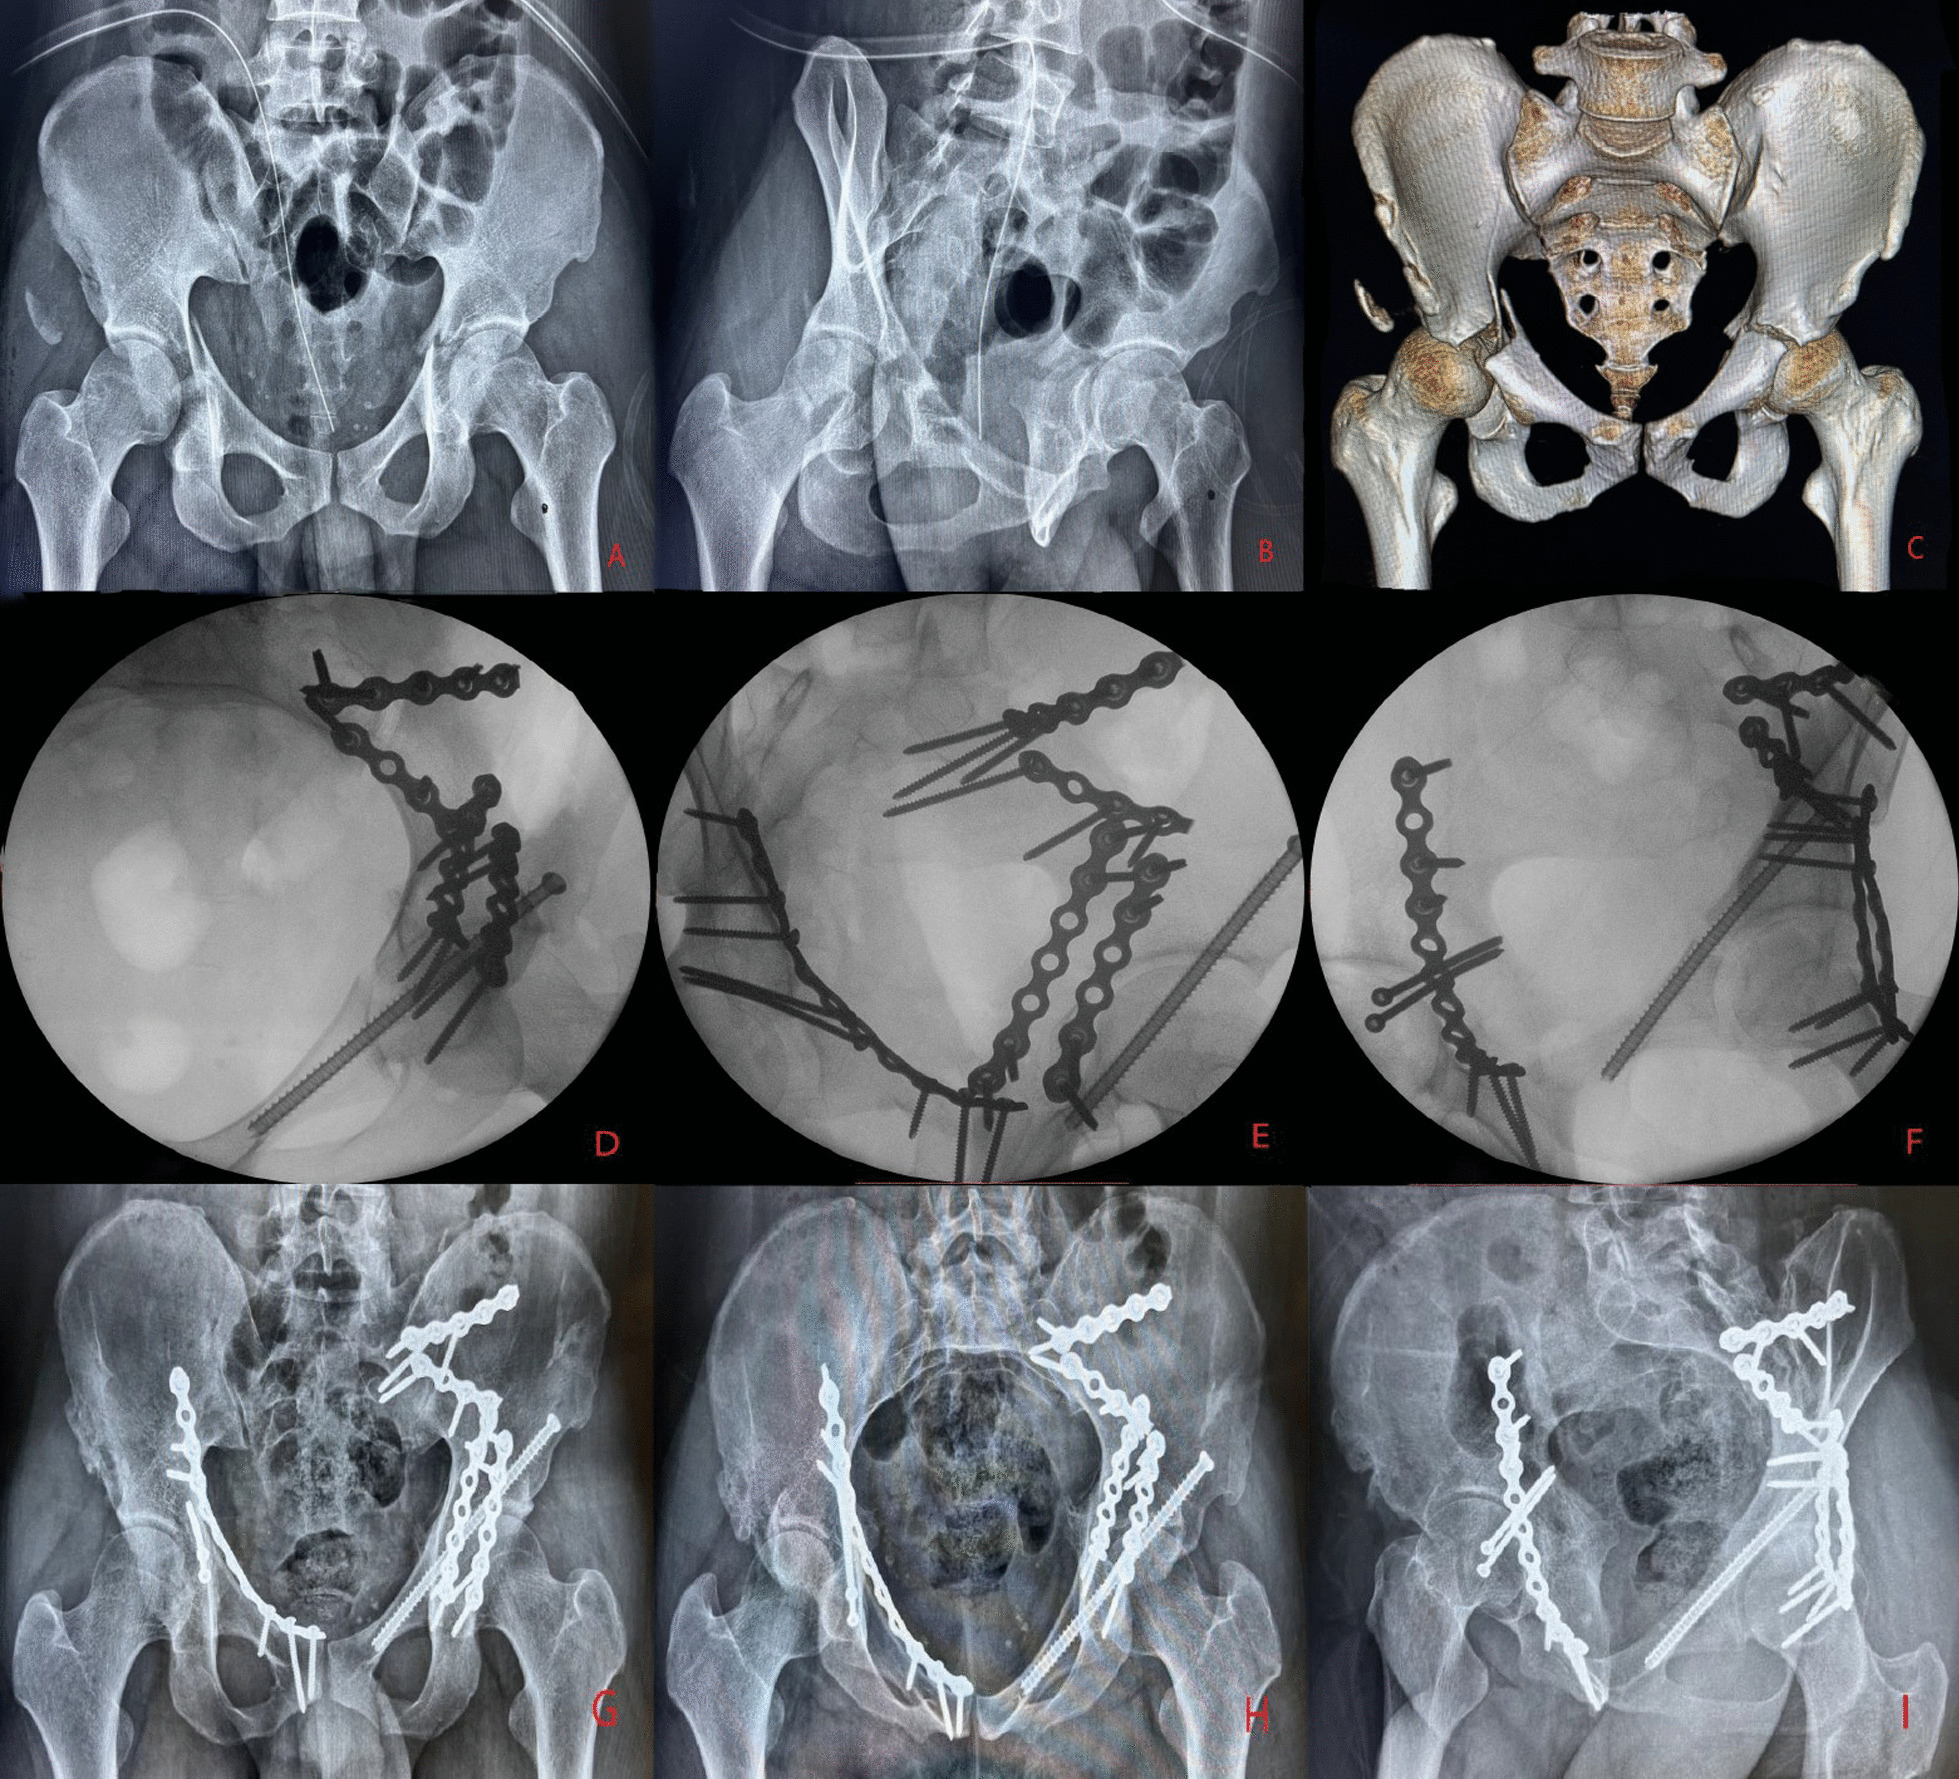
A 25-year-old male patient suffered a Tile C pelvic fracture with bilateral transverse acetabular fractures as a result of a motor vehicle accident and received his first surgical treatment on the 10th day after the injury (A, B, C). The sacroiliac joint was first repositioned in the supine position with a left pararectus approach and fixed with double plates (D). Then, the left acetabulum was repositioned and fixed with the K-L approach (E), and the anterior column of the acetabulum was fixed with an antegrade anterior column screw. Considering that the patient had more intraoperative bleeding, the right pararectus approach was performed to fix the contralateral acetabulum 4 days later (F). The X-ray 1 year after surgery showed anatomical reduction in the pelvis and acetabulum with good fracture healing and no screw breakage or entry into the acetabulum (G, H, I)
